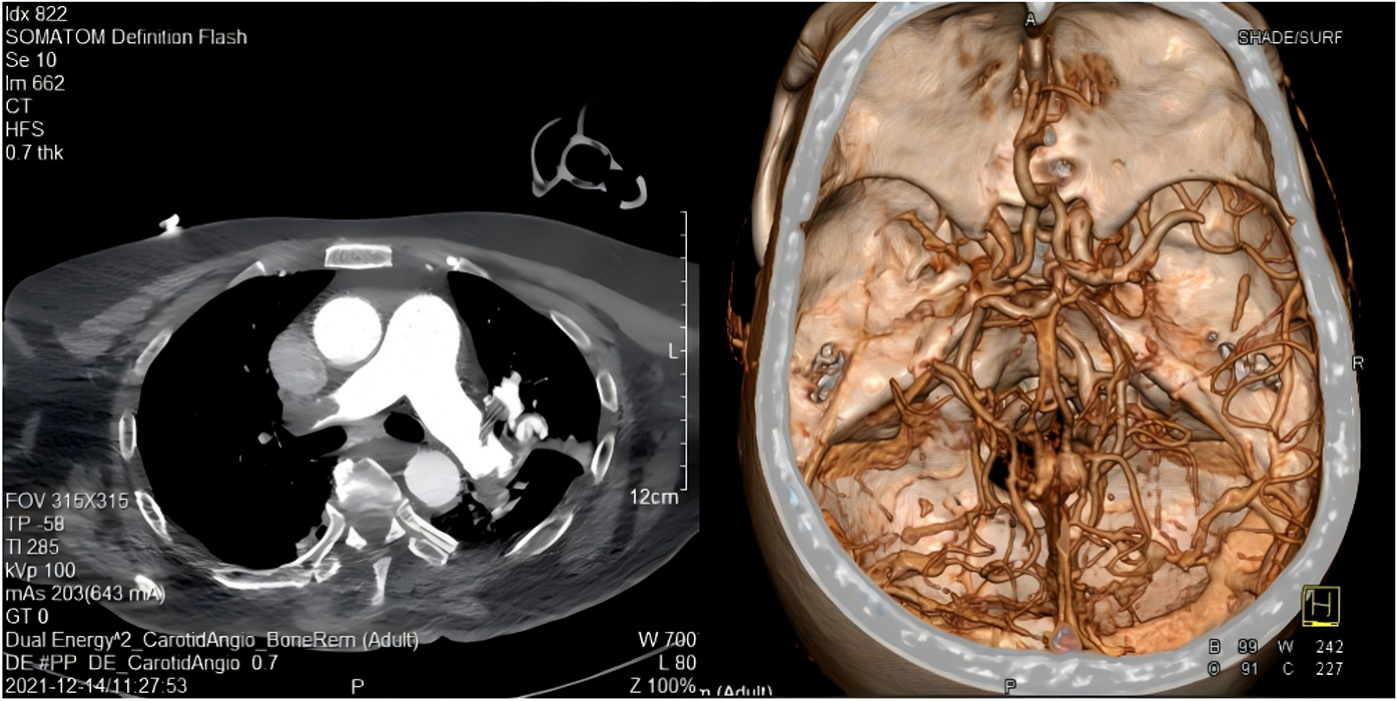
Cervical CTA on December 14, 2021.
